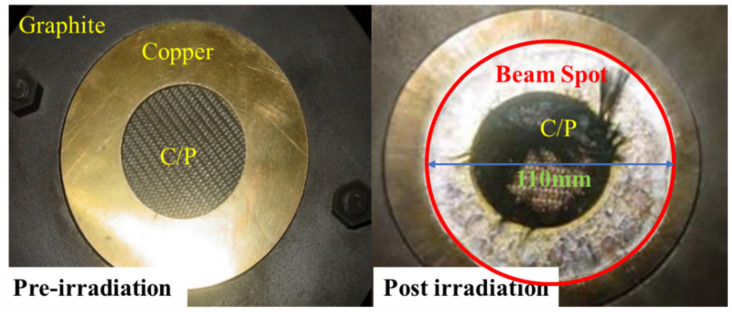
Experimental photos before and after X-ray irradiation.
